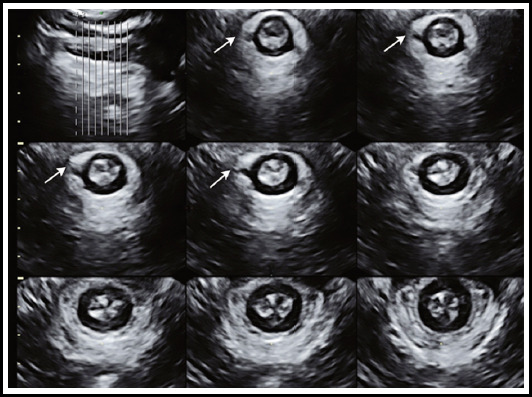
Maternity, 28 years old, 42 days after mild anal incontinence. Perineal three-dimensional ultrasound showed continuous interruption of EAS by perineum), located at 10 o’clock.
